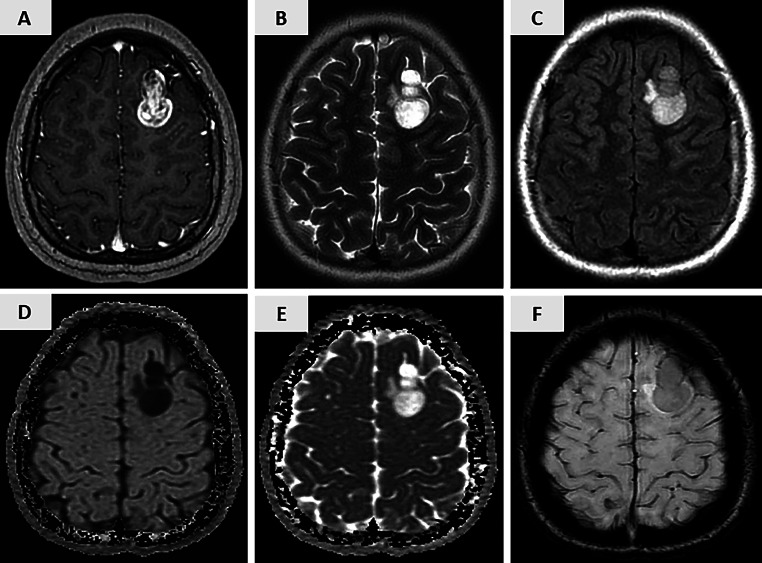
MR imaging characteristics of the left frontal lesion. The lesion abuts the dura mater at its site of apparent implantation and insinuates between the superior and middle frontal gyri, maintaining well-defined margins. (A) Postcontrast T1-weighted image shows strong, homogeneous enhancement. (B) T2-weighted and (C) FLAIR images demonstrate homogeneously high signal intensity with subtle internal signal variation, suggesting variable hydration. There is minimal adjacent white matter edema but no mass effect, consistent with slow growth. (D) Diffusion-weighted imaging (DWI) and (E) apparent diffusion coefficient (ADC) maps show no restriction of water diffusion, supporting the benign, low-cellularity nature of the lesion, consistent with the abundant myxoid matrix and lack of dense cellular packing characteristic of myxomas. (F) SWAN sequence reveals no signal loss, indicating absence of calcification or hemorrhage.•T2-weighted and FLAIR images: Demonstrated homogeneously high signal intensity with subtle internal variation, suggestive of variable hydration (Fig. 2B and C). Minimal perilesional white matter edema was noted, consistent with slow-growing, non-infiltrative behavior.•Diffusion-weighted imaging (DWI) and ADC maps: Showed no restriction of water diffusion (Fig. 2D and E), supporting the benign, low-cellularity nature of the lesion.•SWAN sequence: Revealed no evidence of calcification or hemorrhage.
